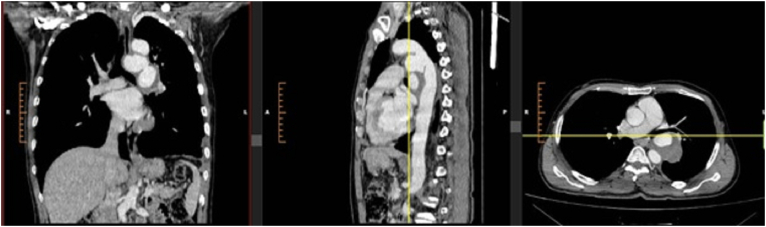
Coronal, sagittal, and axial computed tomography angiography (CTA) demonstrating bilobed pseudoaneurysm of the descending aorta with pressure effects on the distal trachea and left main bronchus.
